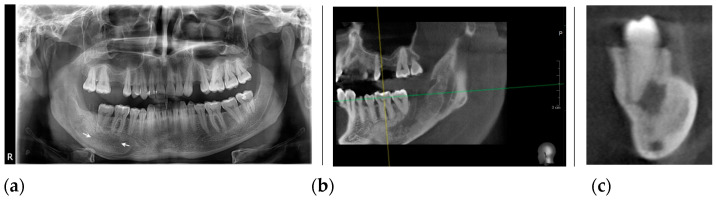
The radiological aspect: (a) Orthopantomography shows the healing process in the ascendant ramus, and the remaining osteolytic lesion at the apical region of the first and second mandibular molars (arrows), one year after marsupialization; (b) The CBCT, sagittal view indicates unmodified bone structure near the molar #46 apices; (c) The CBCT, sagittal view of molar #47 demonstrates the lesion contact and the enlarger of periodontal space.
